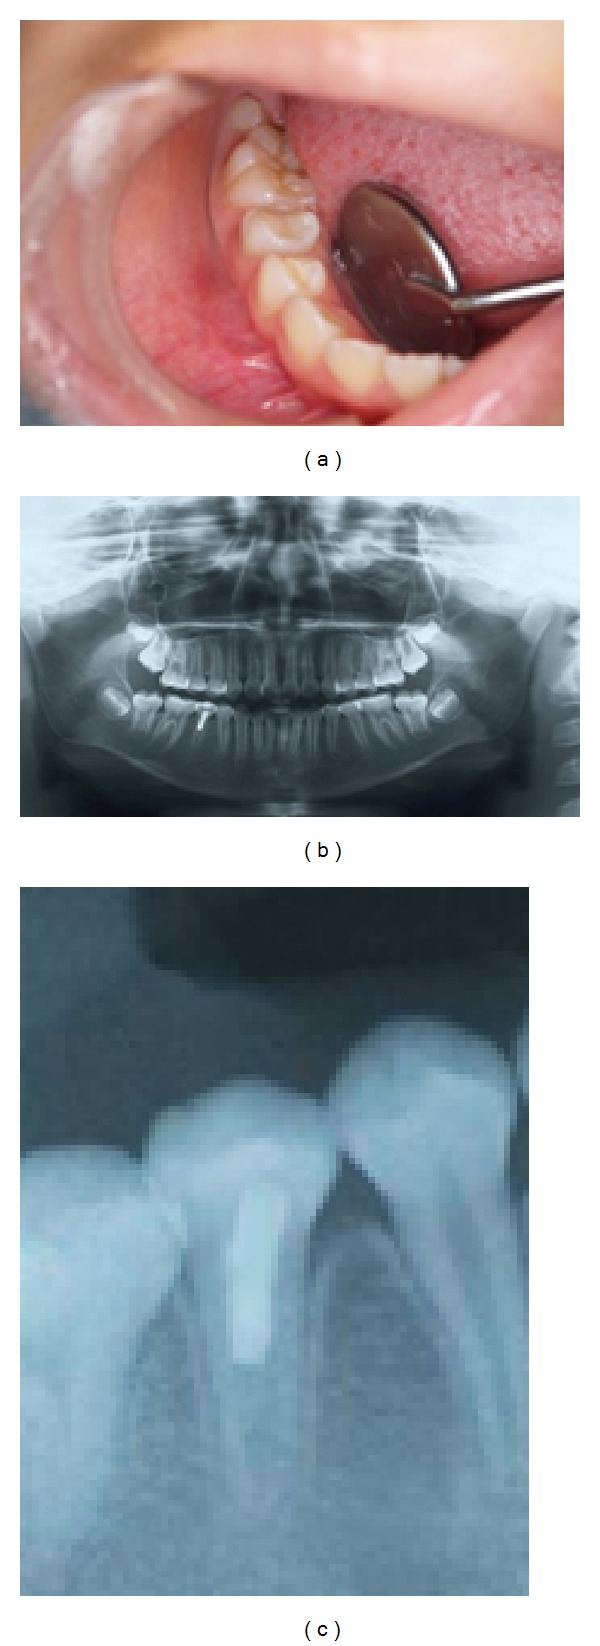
(a) Intraoral photograph showing no abnormalities of gingiva. (b) Panoramic X-ray showing the formation of a dentin bridge and thickening of the canal walls in the mandibular right second premolar. (c) Panoramic X-ray showing the formation of a dentin bridge and thickening of the canal walls and establishment of the periodontal ligament space and lamina dura in the mandibular right second premolar.
